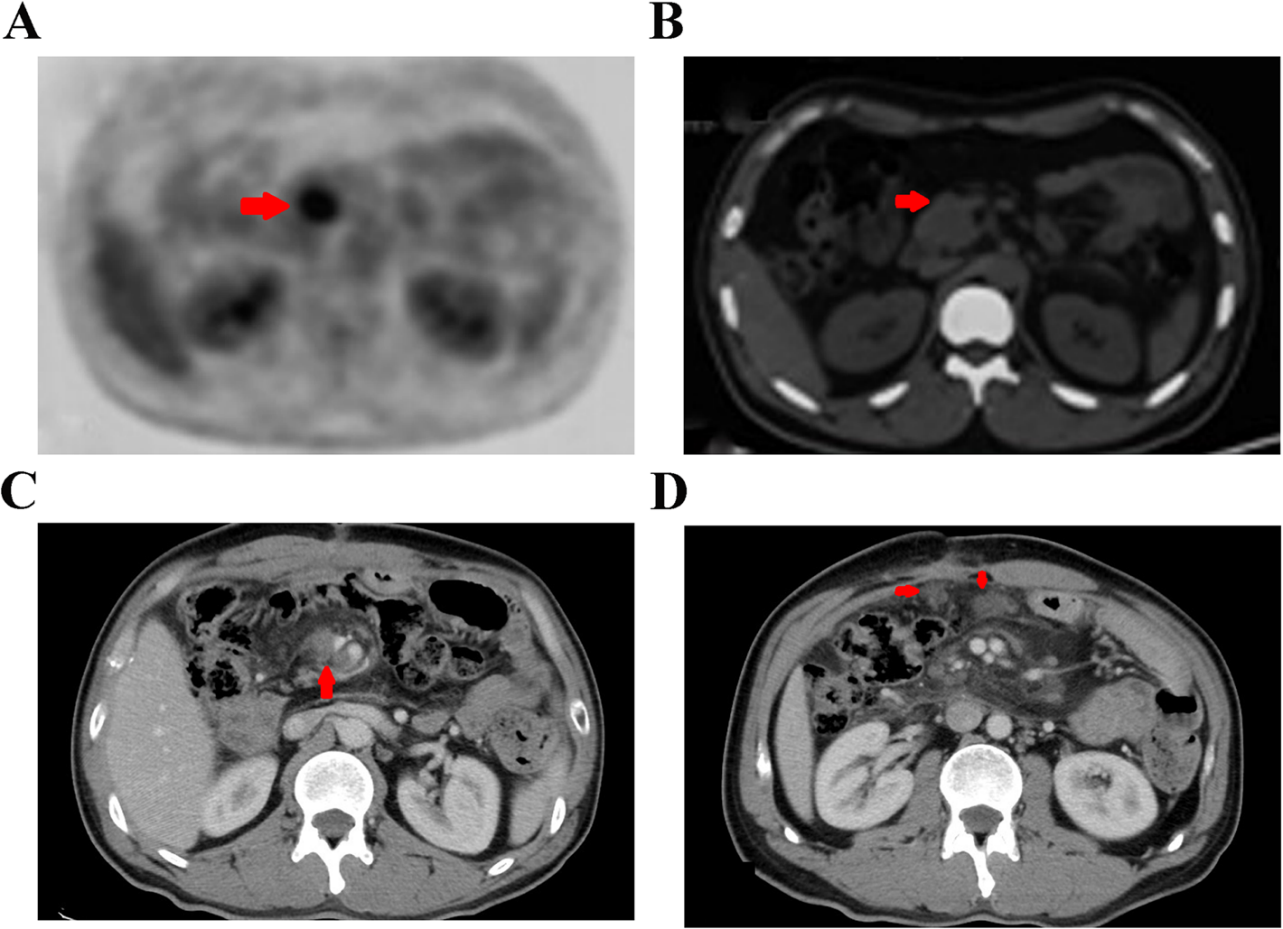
The PET showed high metabolic nodules at pancreatic uncinated process and inclined to be malignant disease at baseline (a, b). The contrasted CT scan showed multiple lymphatic metastases in the mesenteric region(c) and peritoneum (d) beyond termination of adjuvant chemotherapy
